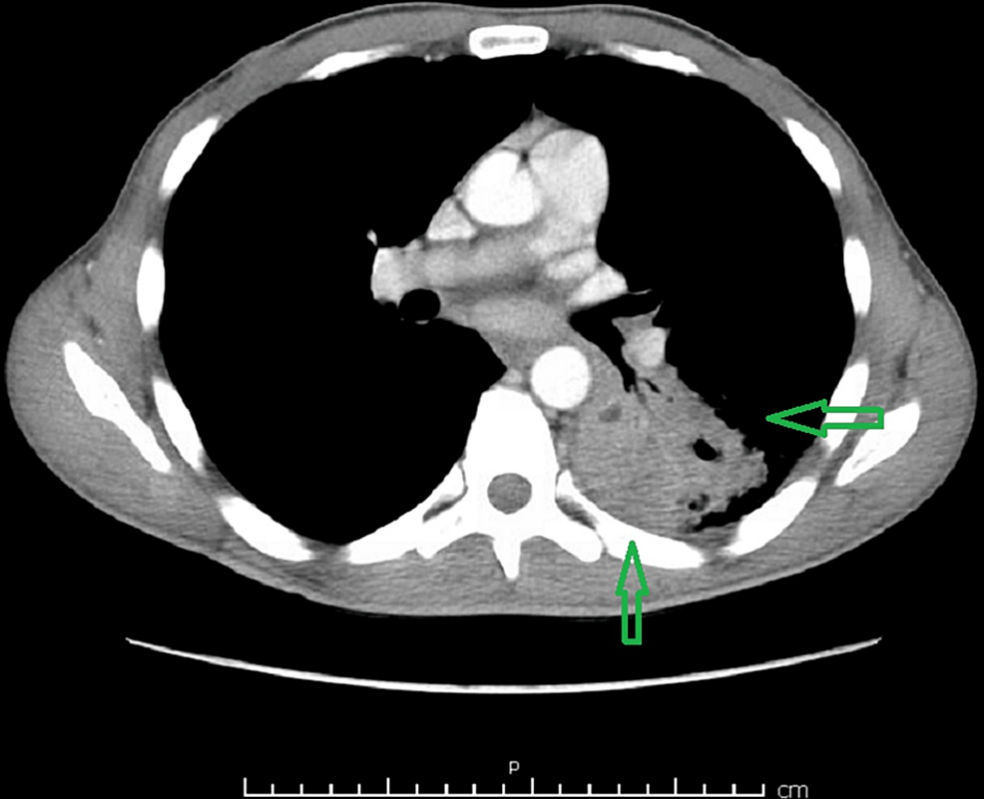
Computed tomography (CT) scan of the chest showing abscess cavity internal fluids or debris as shown with green arrows.
